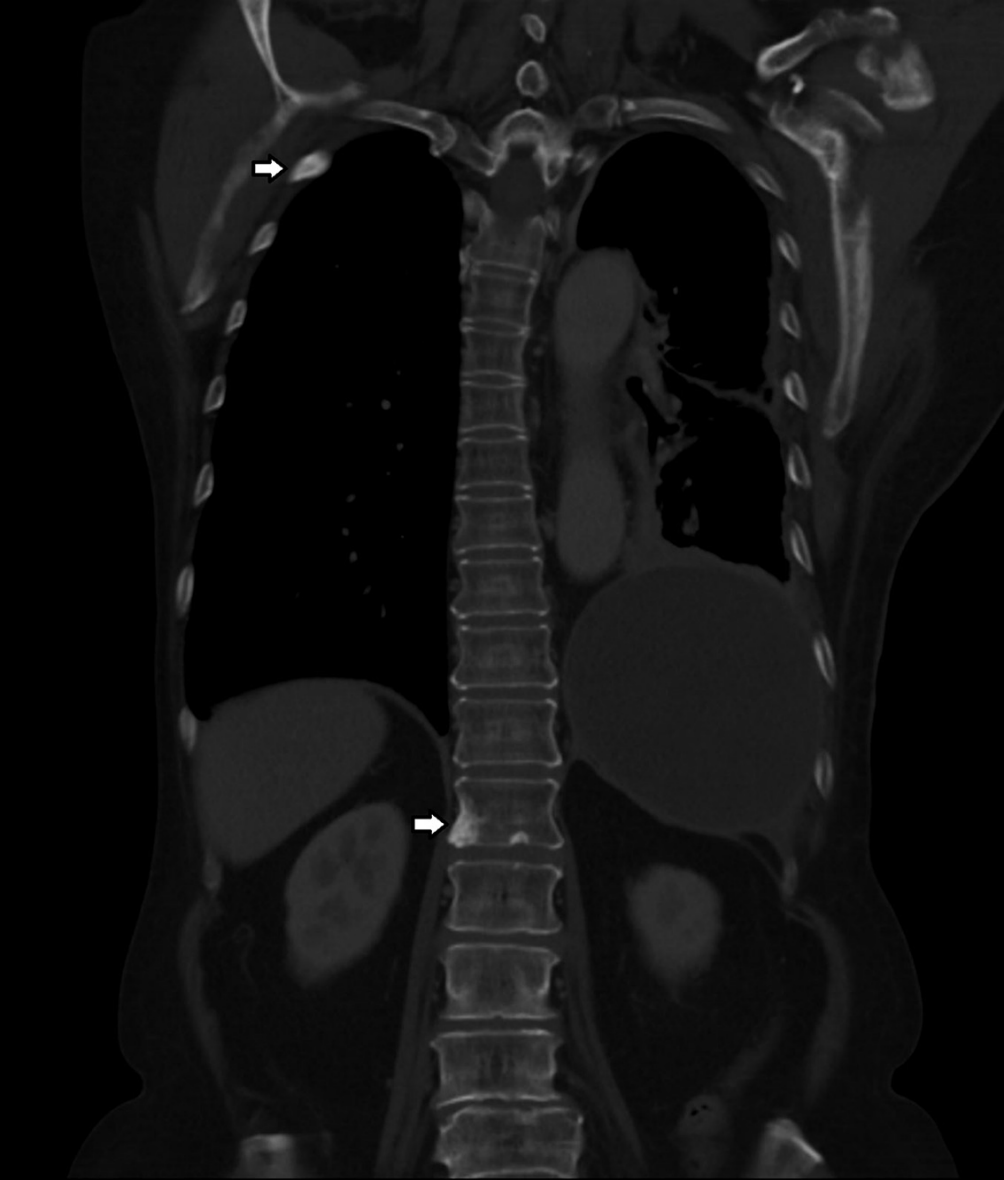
CT scan in the bone window showed sclerotic bony metastases to the ribs and spine.
